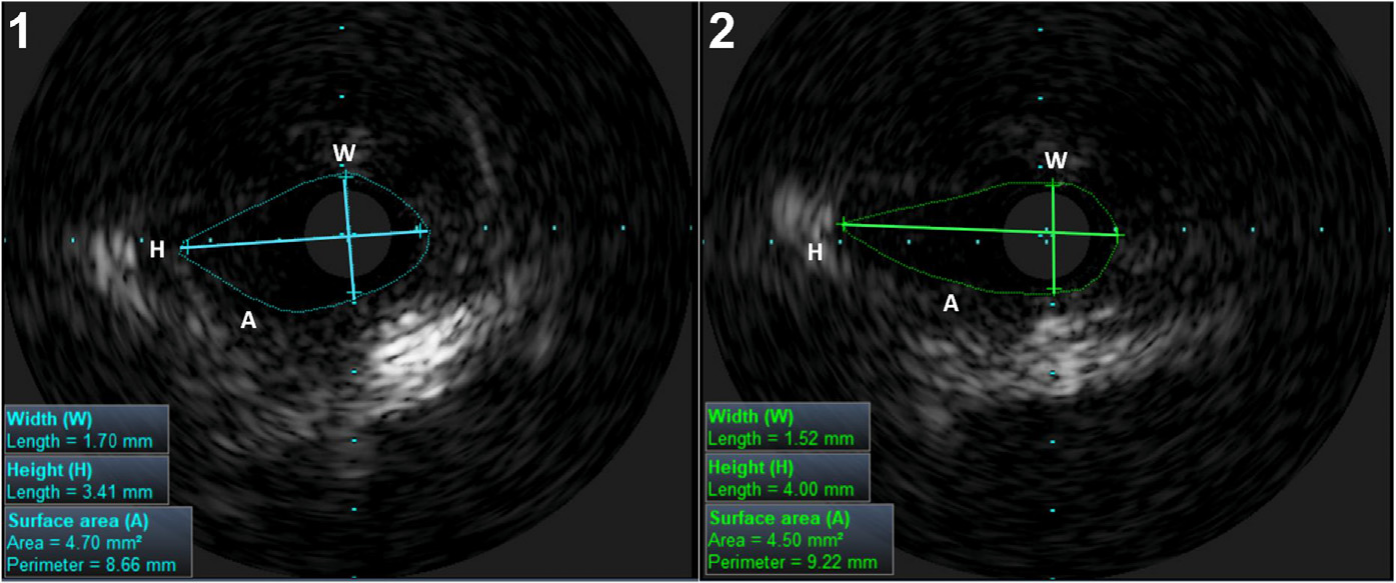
Intravascular ultrasound (IVUS) analysis approach. IVUS measurements of the height (H), width (W), and coronary surface area (A) at baseline (1) and during adrenaline-induced stress (2) for the assessment of the “width/height ratio” to determine the coronary vessel shape at baseline, the “width/height ratio during adrenaline-induced stress”/“width/height ratio at baseline,” to evaluate external compression and to estimate the change in the coronary surface area.
